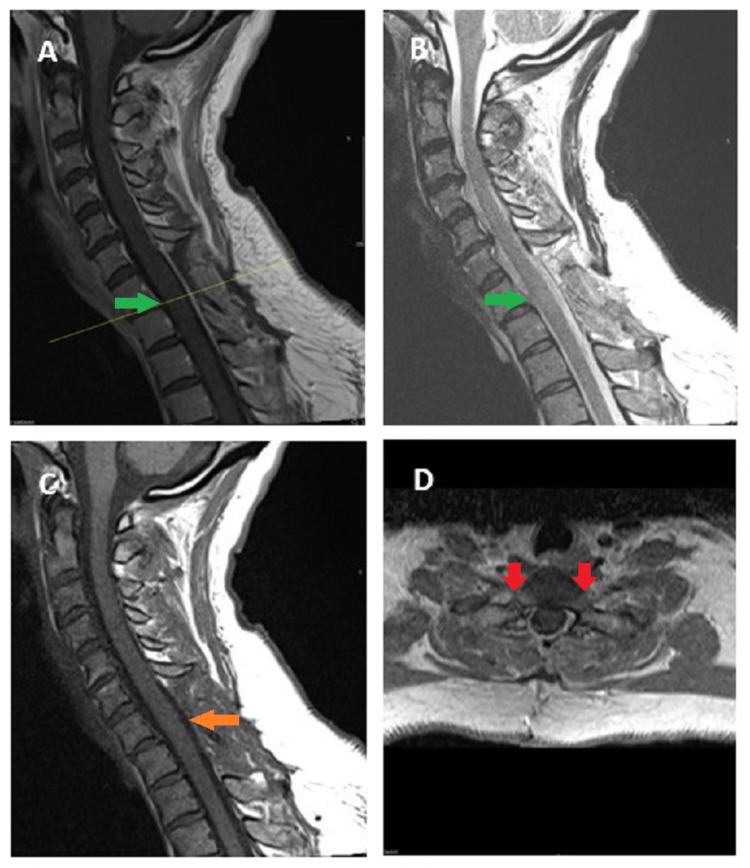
Post-Operative MRI after C7-T1 Laminectomies and Partial bilateral C7-T1 Facetectomies with Resection of Lesion (A) Sagittal T1-weighted MRI showing that the previously seen narrowed dural sac at C7-T1 related to the bilateral extradural foraminal lesions was decompressed (green arrow); (B) Sagittal T2-weighted MRI again shows interval resection of bilateral neural foraminal rim enhancing extramedullary lesions and decompression of severe lateral spinal cord compression seen on prior MRI (green arrow); (C) Sagittal postcontrast T1-weighted MRI shows a small amount of abnormal signal in dorsal spinal cord at C7-T1, likely residual edema related to the relieved spinal cord compression (orange arrow); (D) Axial postcontrast T1-weighted MRI shows no remaining bilateral foraminal nerve root compression at C7-T1 (red arrows)
